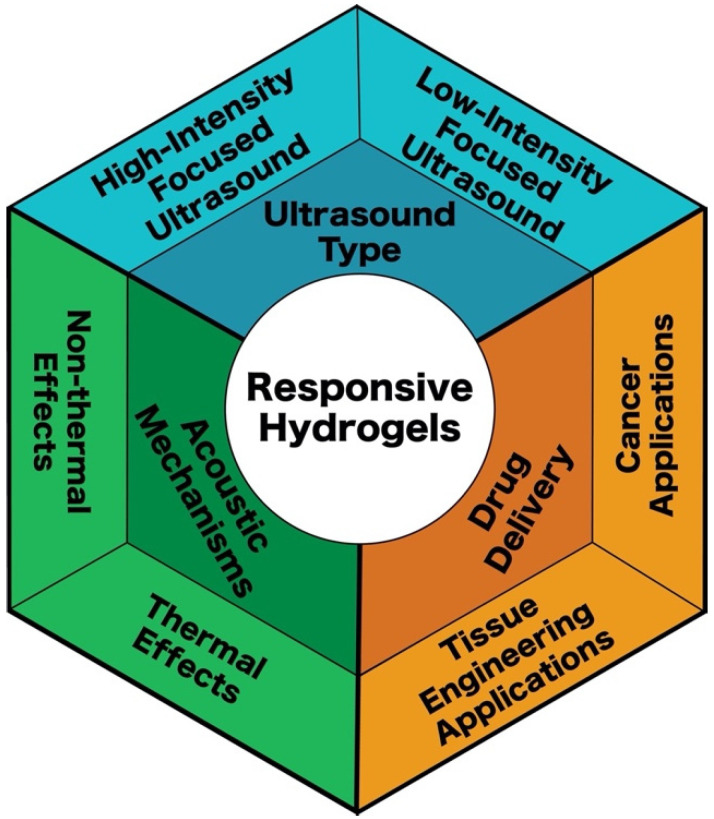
Ultrasound-sensitive hydrogels are designed to respond to ultrasound (either low or high-intensity) via thermal or non-thermal effects. Applications for these drug delivery systems include cancer therapy and tissue engineering.
